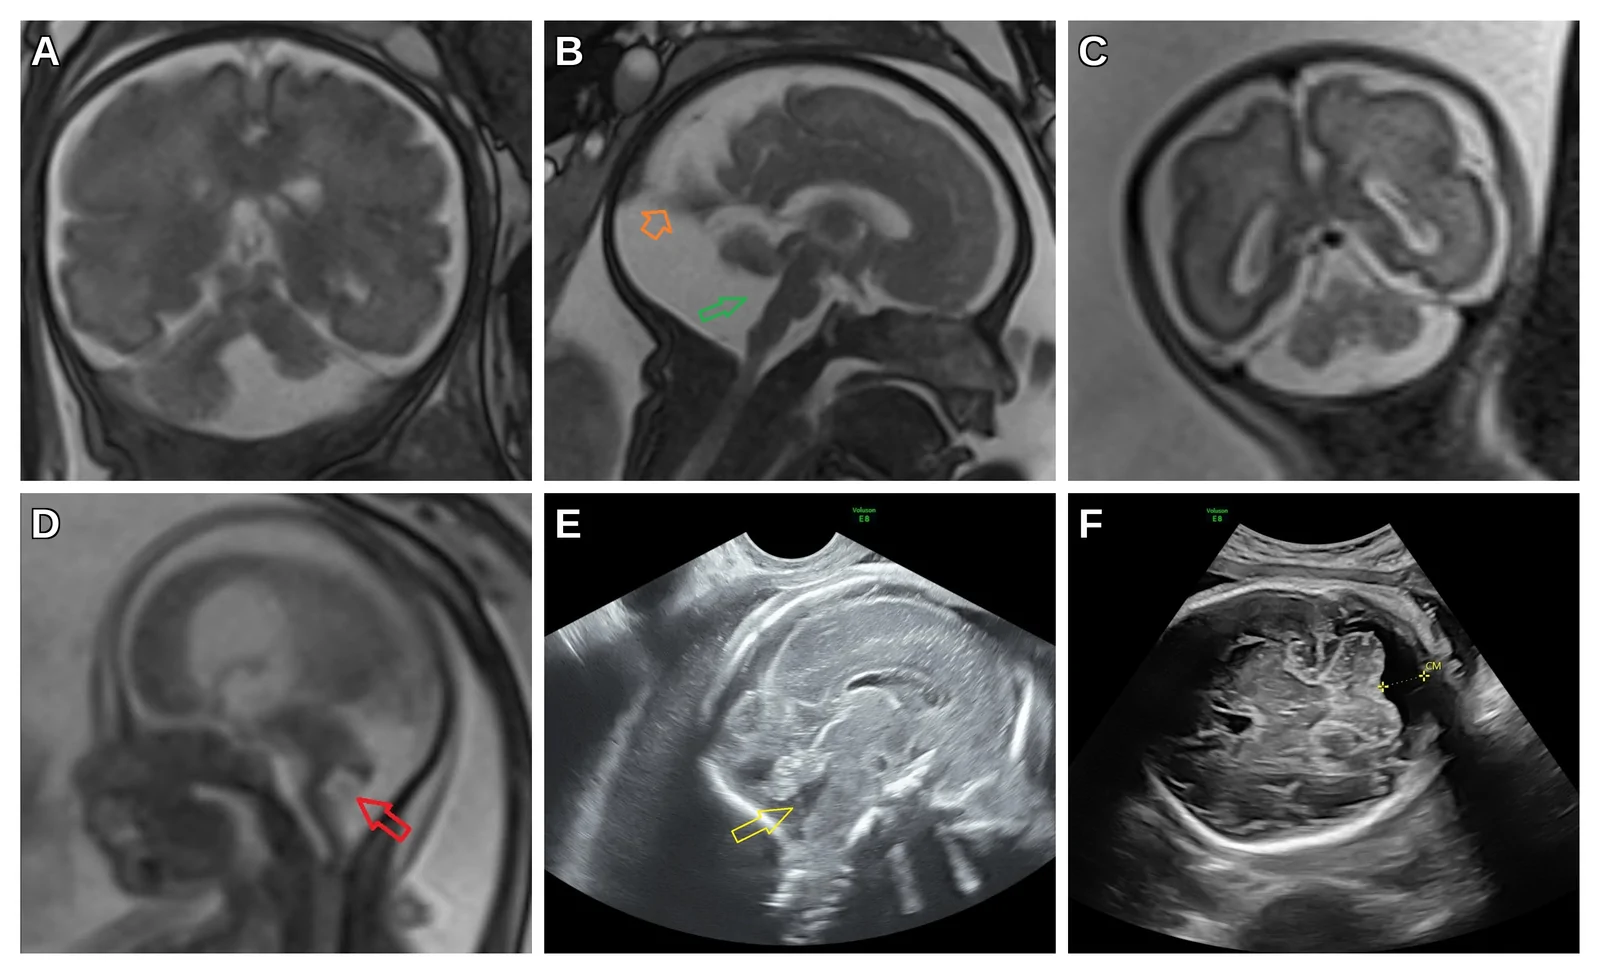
(A): Dandy–Walker malformation at 31 weeks. In fetal MRI, the separated cerebellar hemispheres are clearly visible in the transcerebellar section. (B): In the same case, torcular elevation (green arrow) and upward rotated hypoplastic vermis (orange arrow) are major characteristics of the anomaly. (C): Cerebellar hypoplasia at 31 weeks. (D): Walker–Warburg syndrome at 20 weeks shows cerebellar and brainstem hypoplasia (red arrow). (E): An increased tegmentovermian angle was observed on prenatal ultrasonography in a 23-week-old patient with a Blake’s pouch cyst (yellow arrow). (F): In a case of Mega Cisterna Magna at 28 weeks: prenatal ultrasonography revealed an enlarged cisterna magna in the transcerebellar axial plane.
